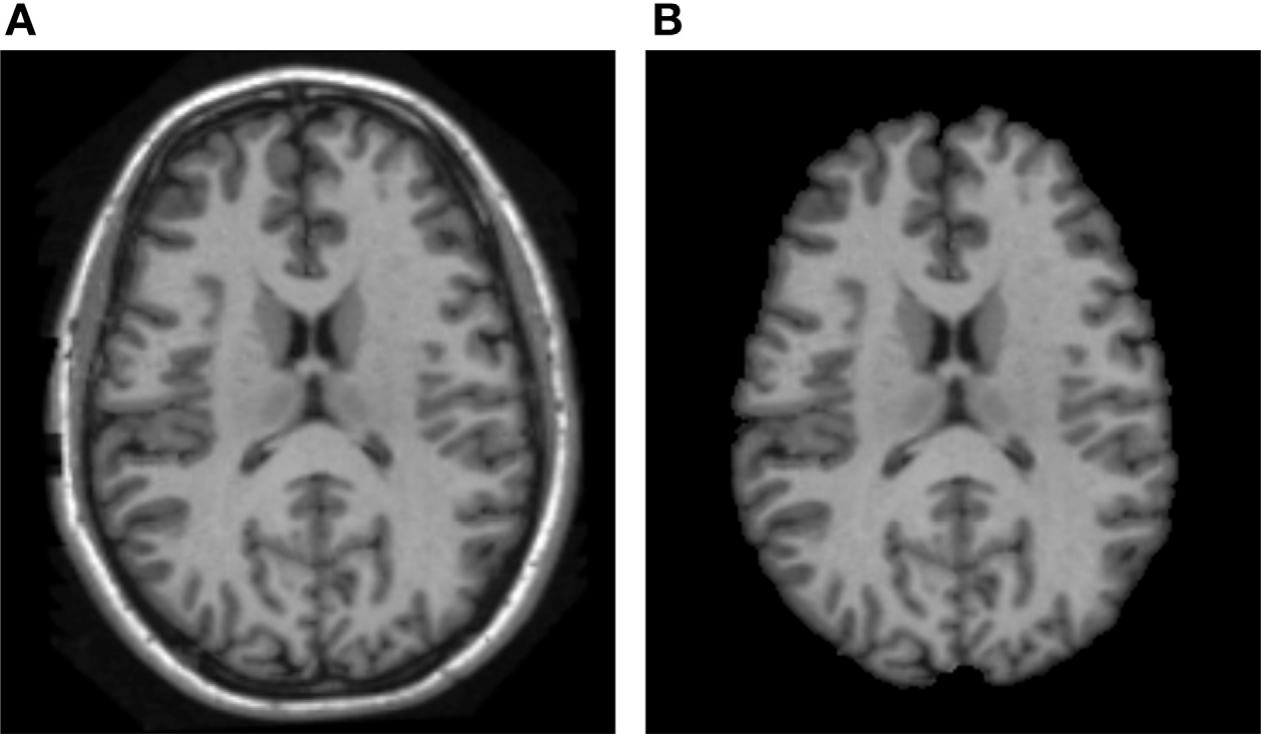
ABC based brain skull-stripping result. (A) the brain tissue in original MRI scan, (B) the skull-stripped brain.
